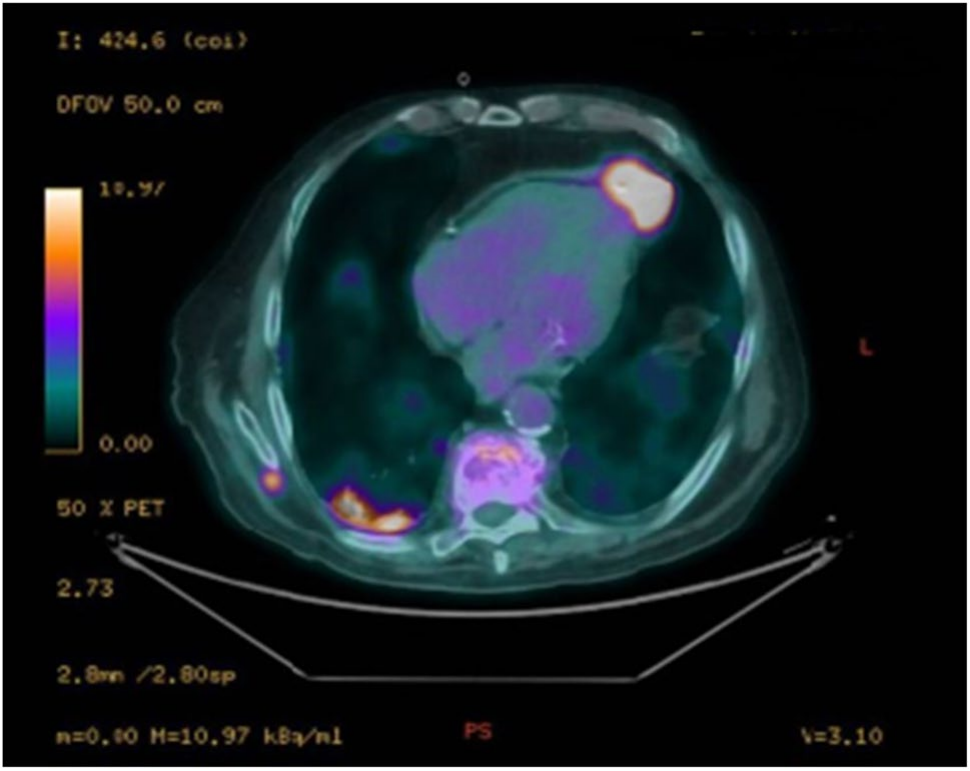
Images with hybrid technique with positron emission tomography-computed tomography (digital PET-CT) with 18F-FDG (219 MBq e.v.). The technique shows the presence of the collection localized near the cardiac apex, characterized by ring-shaped uptake (SUVmax 15.7)
